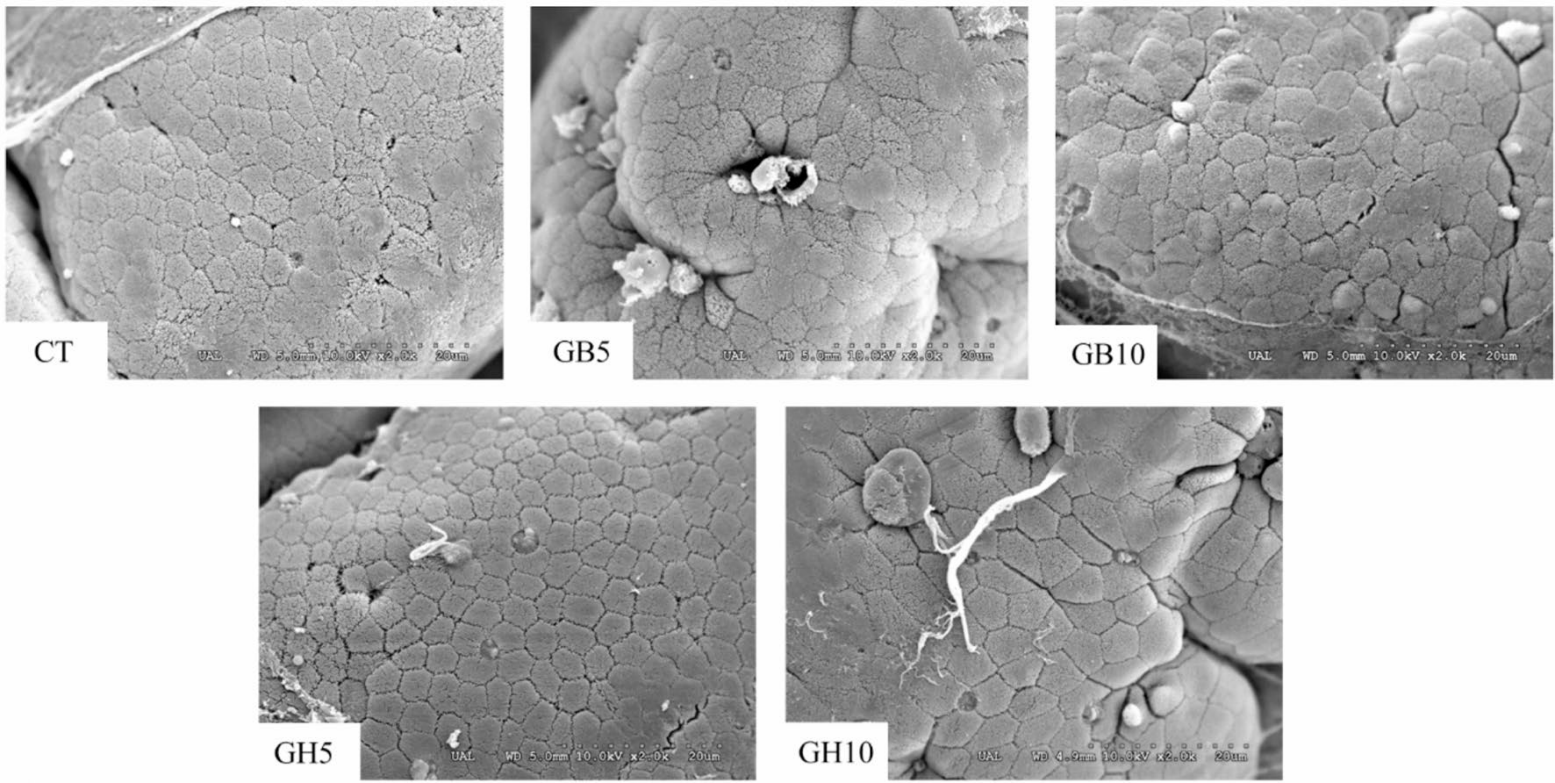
SEM details of the enterocyte apical surface in gilthead seabream juveniles fed the different experimental diets (SEM. Scale: 20 μm). Dietary codes: CT: control; GB5: 0.5% inclusion of LB-GREENboost; GB10: 1% inclusion of LB-GREENboost; GH5: 0.5% inclusion of LB-GUThealth; GH10: 1% inclusion of LB-GUThealth.
